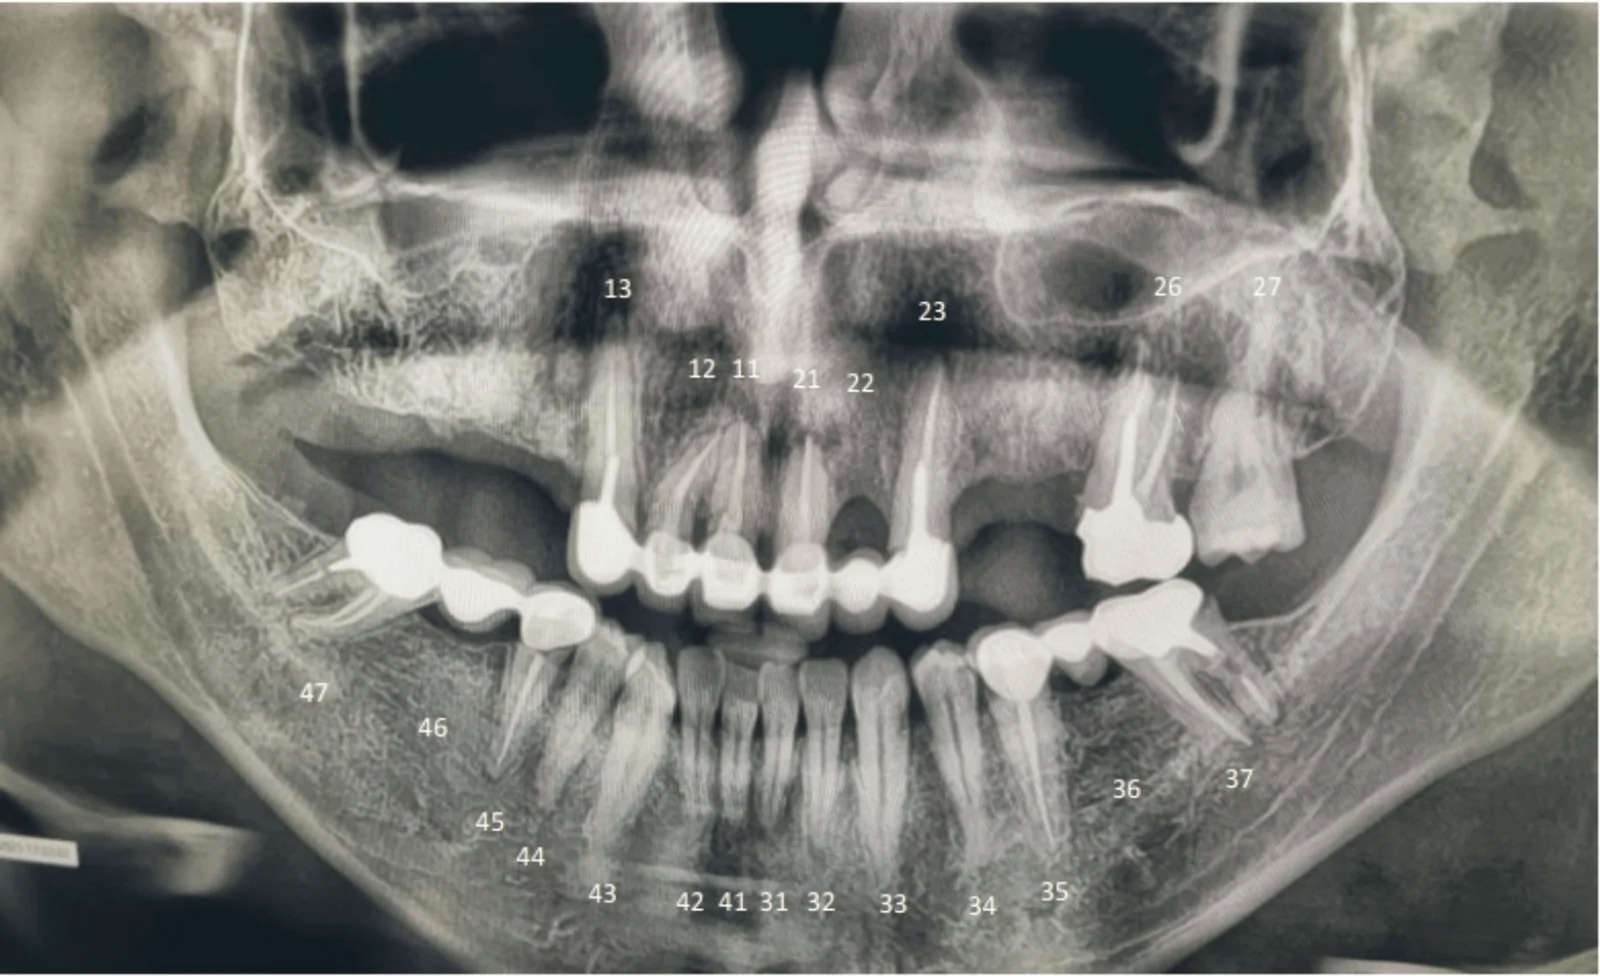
Panoramic radiograph taken after two years of follow-up with fixed prostheses showing the stability of the deep periodontium
